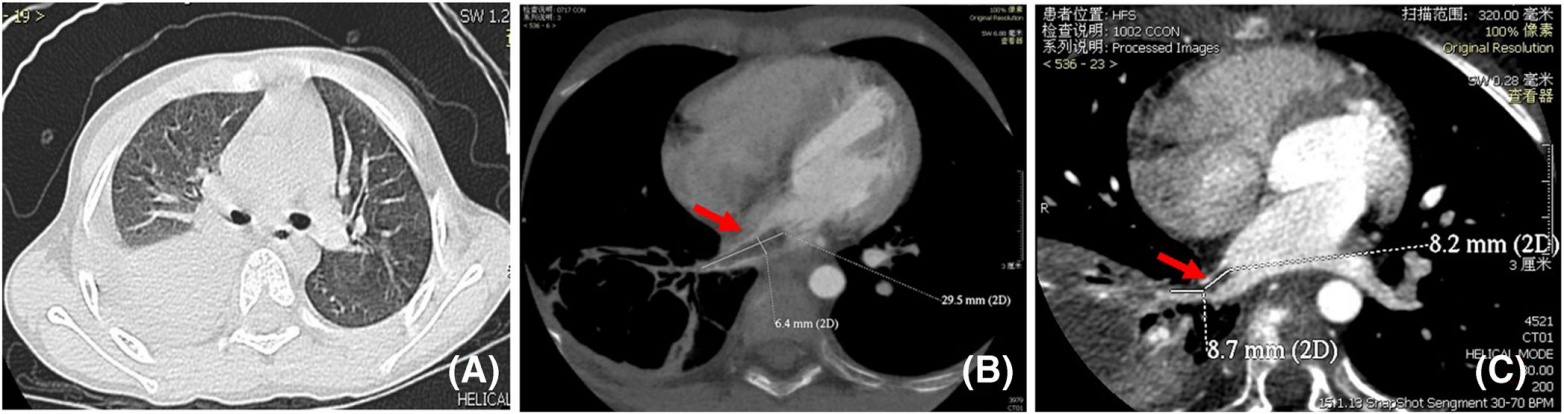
(A) CT showed consolidation of the right lower lung. (B) CTPA revealed a filling defect in the right pulmonary vein that extended to the left atrium; (C) the filling defect shrank after treatment
